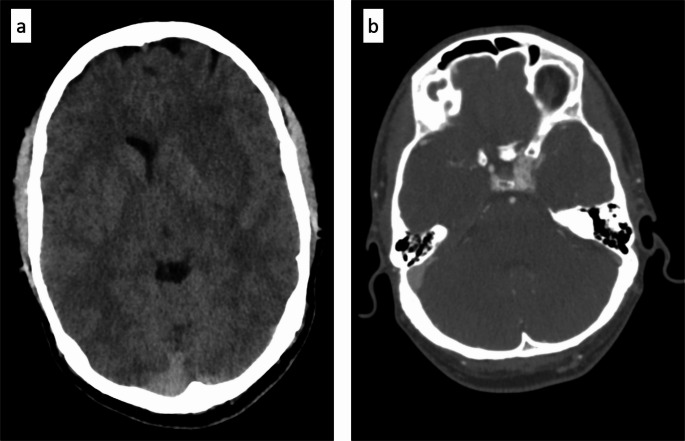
NECT at admission showed a mainly hypodense lesion located at the left lentiform nucleus and external capsula (a). CTA showed increased contrast in the left cavernous sinus (b)
